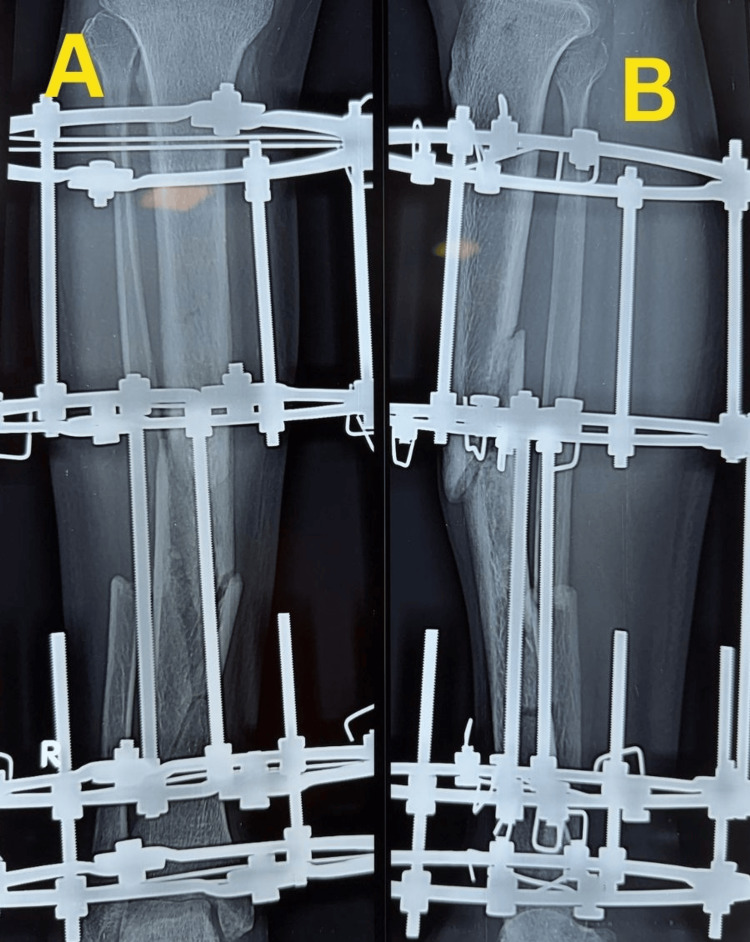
Follow-up radiographs showing progressive consolidation Follow-up radiographs obtained on 11 March 2026 demonstrating progressive consolidation and progression toward union: anteroposterior view (A) and lateral view (B).
